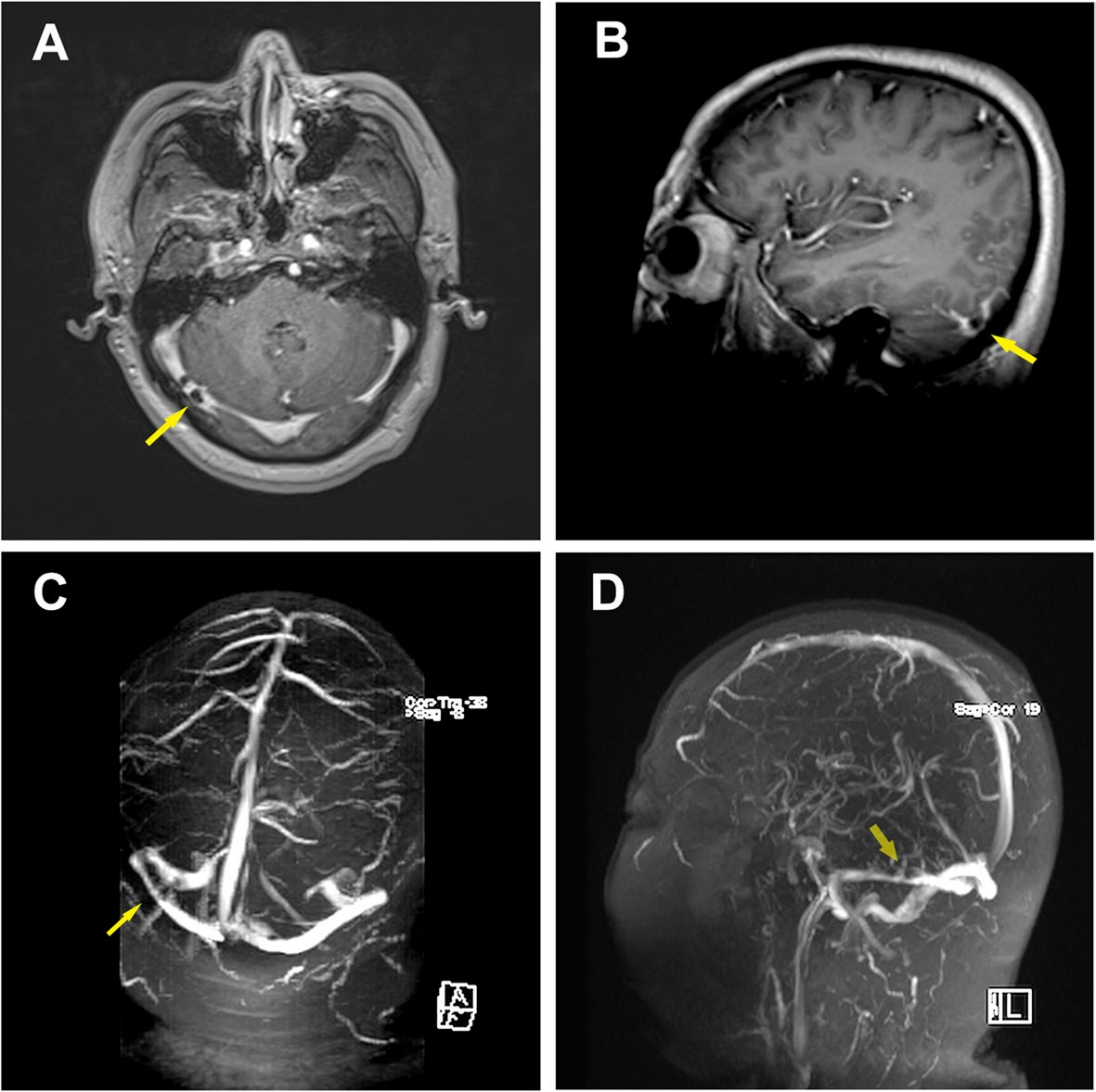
A and B Contrast-enhanced magnetic resonance imaging revealed the suspicious filling defect of the right transverse sinus, and the larger one (*arrow*) was about 6 mm, suggesting the possibility of thrombosis in the right transverse sinus. C and D Magnetic resonance venography imaging showed multiple filling defects in the right transverse sinus and sigmoid sinus (*arrow*), and the luminal visualization was slender than that on the left (*arrow*), suggesting multiple thrombosis in the right transverse sinus and sigmoid sinus
